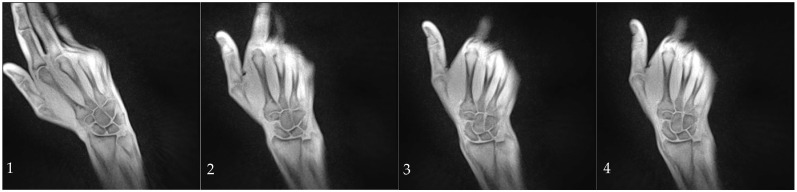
Series of images captured from radial (1) to ulnar deviation (4) during the abduction/adduction maneuver. These images were acquired using a T1-weighted rtMRI sequence with iterative reconstruction, achieving a temporal resolution of 50 ms. Images (1–4) correspond to frames 156, 207, 226, and 262 out of a total of 300 frames.
